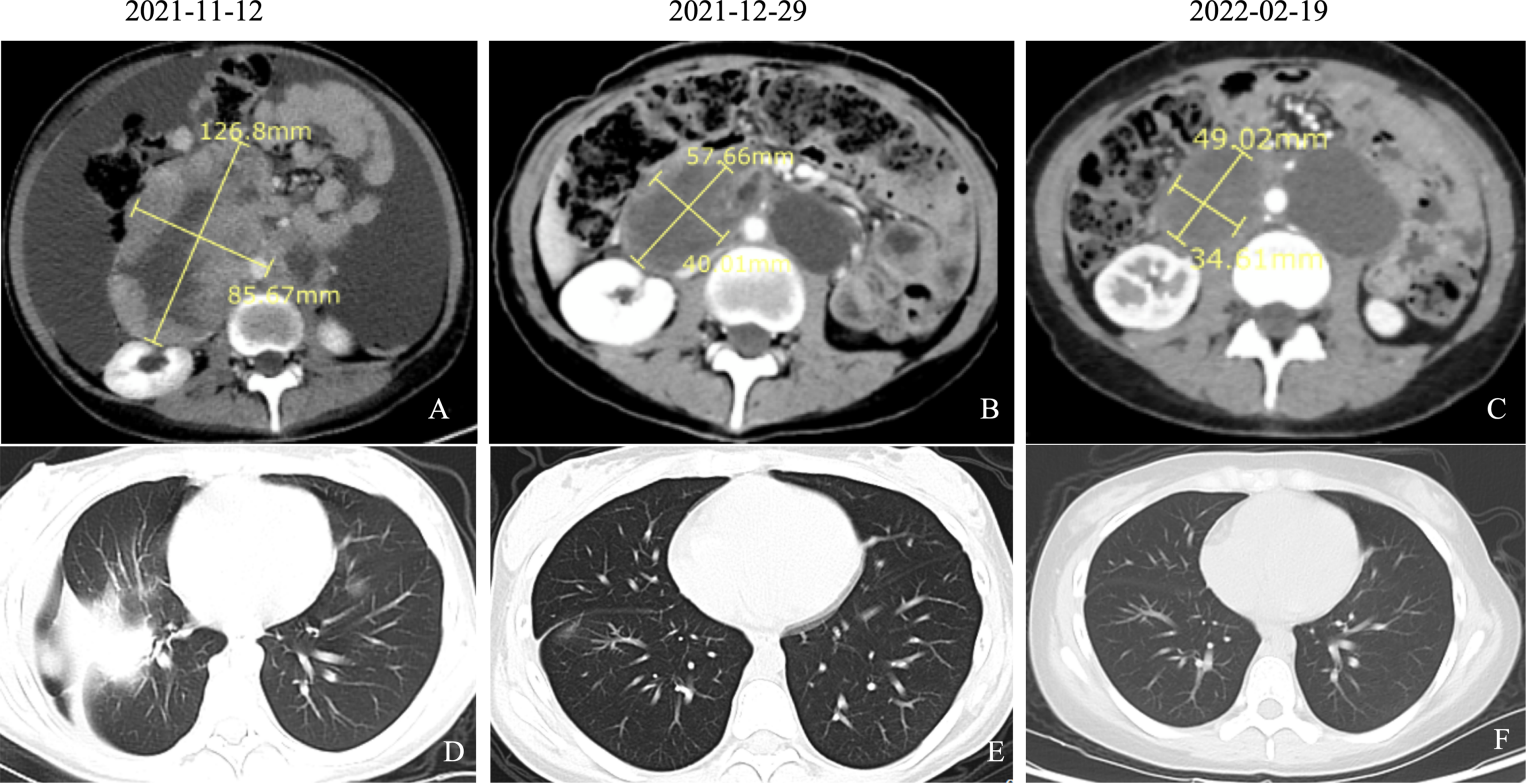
CT findings of pelvic sarcomas and lung metastasis over the course of patient’s treatment. (A) Abdominal CT scan (November 12, 2021) showed abdominal and pelvic lesions, blurred surrounding intestinal fat space, and multiple enlarged lymph nodes behind the peritoneum, which were similar to the previous findings (October 20, 2021). Abdominal and pelvic effusions were more advanced than before. (B) Abdominal CT scan (December 29, 2021) showed abdominopelvic space occupation, blurred surrounding intestinal fat space, multiple enlarged lymph nodes in the retroperitoneum, and lesions smaller than previously observed (November 12, 2021). (C) Abdominal CT scan (February 19, 2022) showed abdominopelvic multiple occupancy, blurring of surrounding intestinal fat spaces, multiple enlarged lymph nodes in the retroperitoneum, and partial reduction of previous (December 29, 2021) lesions. (D) Chest CT scan (November 12, 2021) showed right pleural effusion with atelectasis, right pulmonary nodule, and possible metastasis. (E) Chest CT scan (December 29, 2021) showed the right pulmonary nodules disappeared compared to the previous ones, and new nodules were observed (possible metastasis). (F) Chest CT scan (February 19, 2022) showed the right pulmonary nodule disappeared compared with the anterior part.
